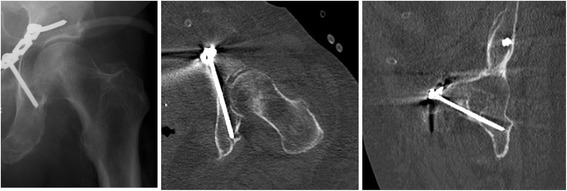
Radiographs showing an ideal position of the infra-acetabular screw
